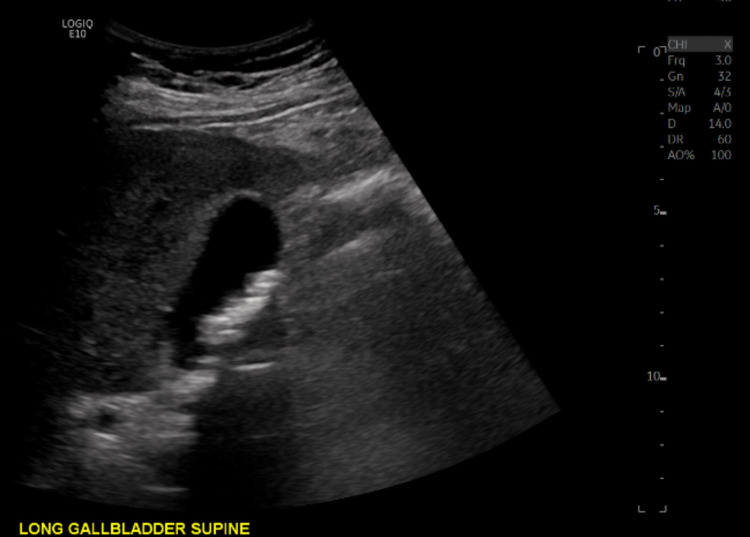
Liver ultrasound showing multiple, small mobile gallstones with no suspicious gallbladder wall thickening or pericholecystic fluid.
